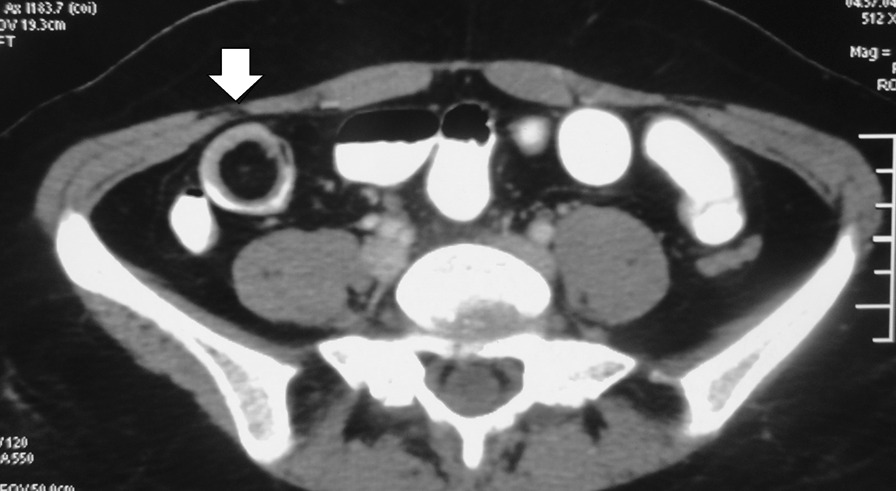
Computed tomography image (transverse plane) showing classical target sign (arrow)
